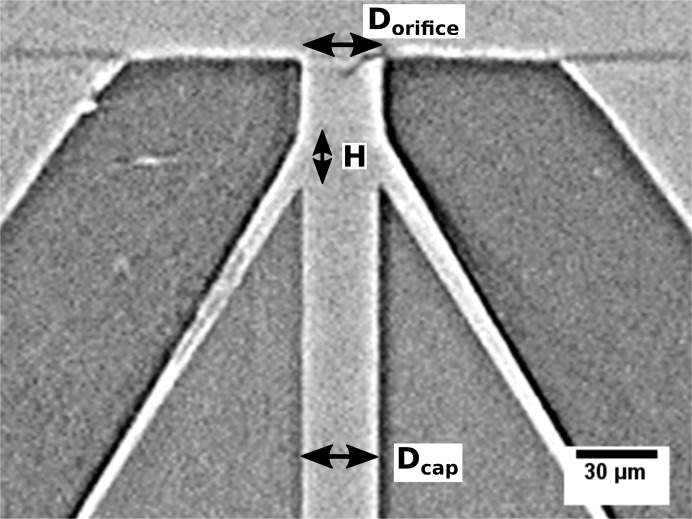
A slice through an X-ray tomogram of the nozzle used in the FLASH experiments. The nozzle is almost cylindrical/symmetric and consists of a sharpened glass capillary with an inner bore diameter of 30 µm surrounded by an injection-molded ceramic conical piece. The capillary transports the sample liquid to the nozzle exit where a free-standing liquid jet is formed by gas flowing through the interstitial space and out through the orifice. Tomography was performed with 25 keV photon energy at the P05 endstation at the PETRA III synchrotron facility.
